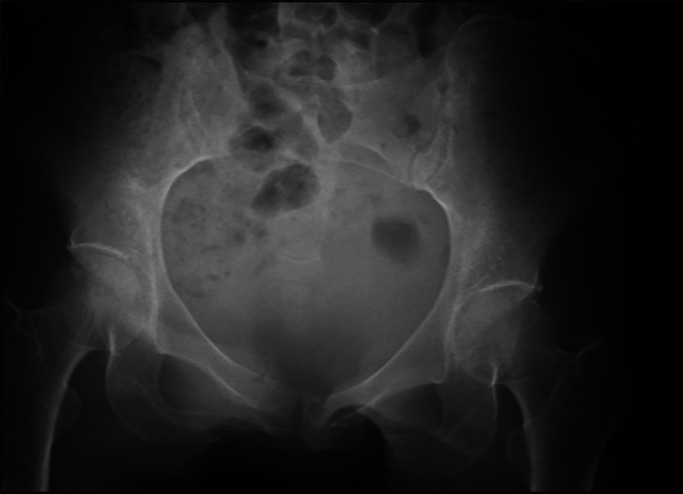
X-ray of pelvis at nearby hospital.
